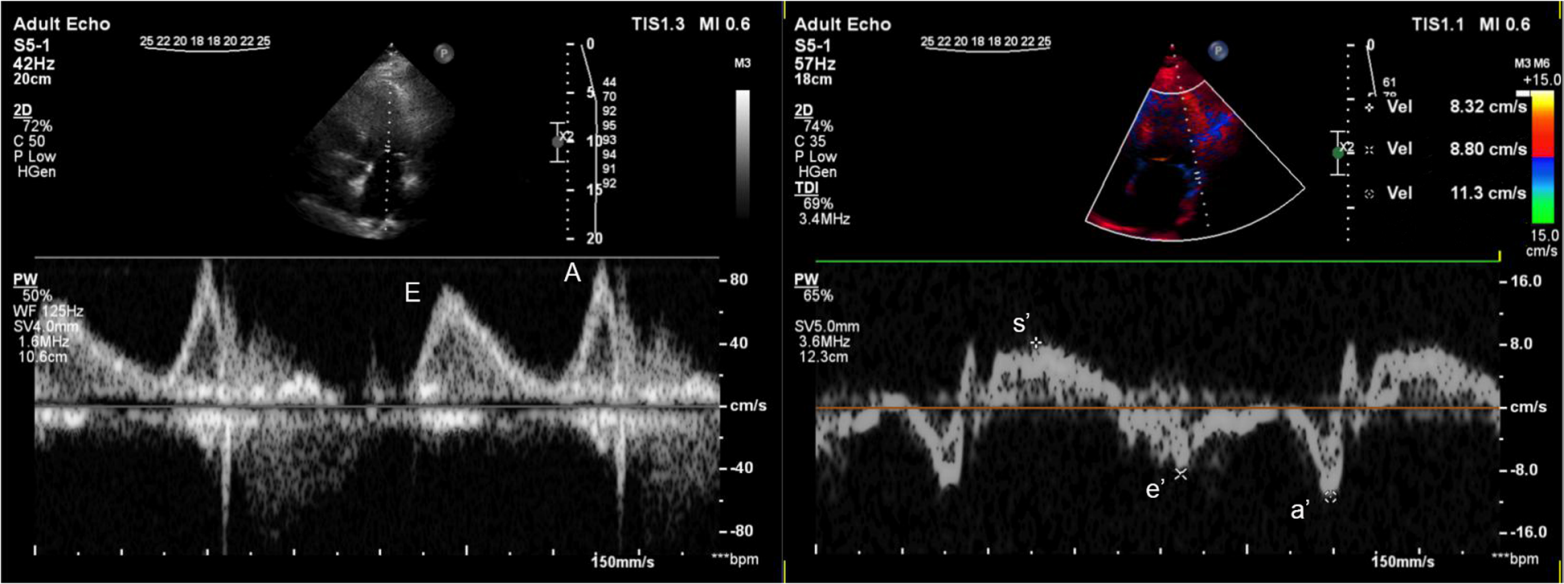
Representative echocardiographic results in polyphasic group after 2 years follow-up. Representative images of echo patterns of mitral inflow from the 4-chamber view in the left panel: impaired relaxation pattern with decreased E wave velocity and increased A wave velocity. Typical tissue Doppler image of the lateral LV wall on the right: decreased lateral s′ (systolic velocity), decreased lateral e′ (early diastolic myocardial relaxation velocity) and increased lateral a′ (late diastolic myocardial relaxation velocity)
